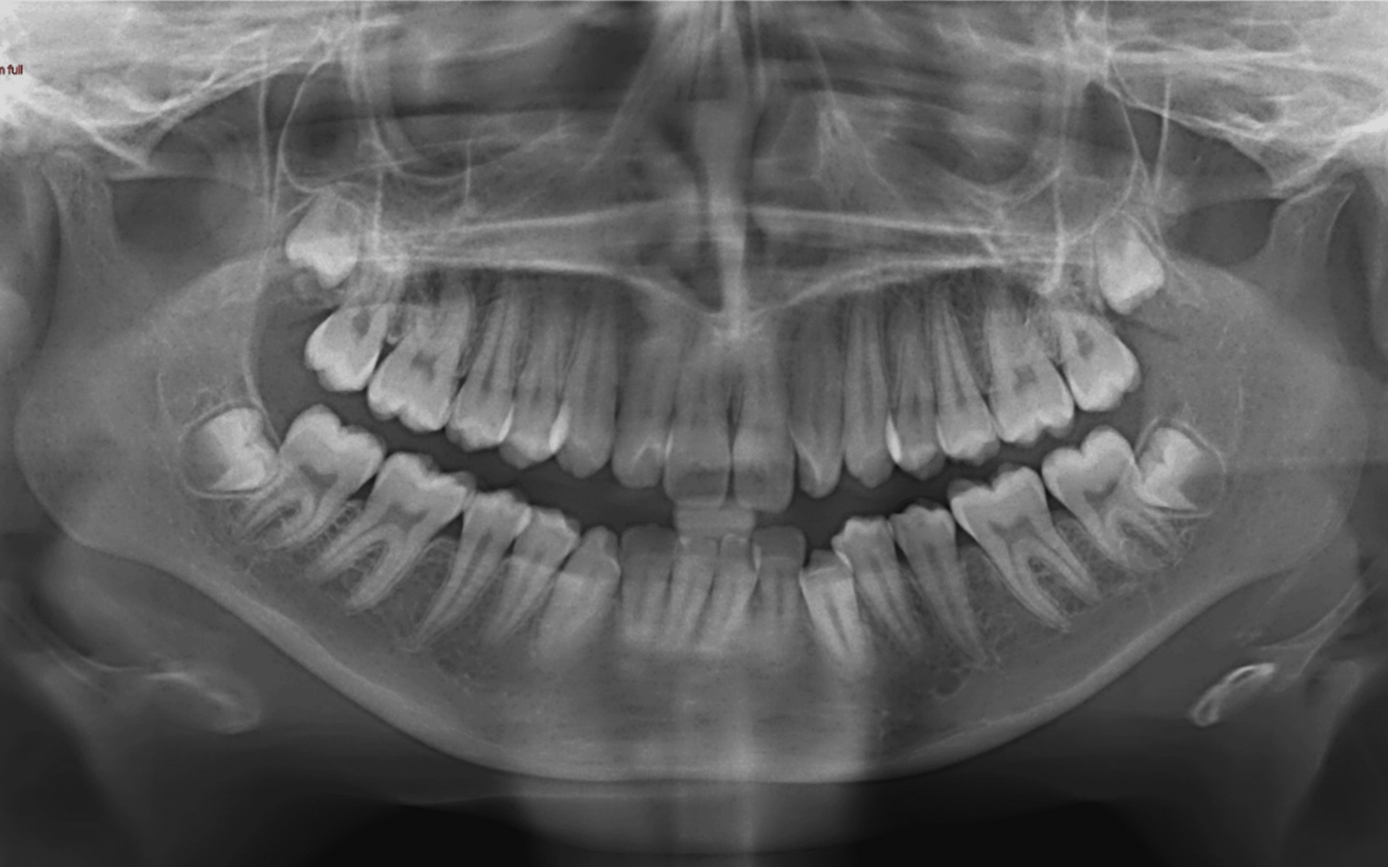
Pre-treatment dental panoramic radiograph
